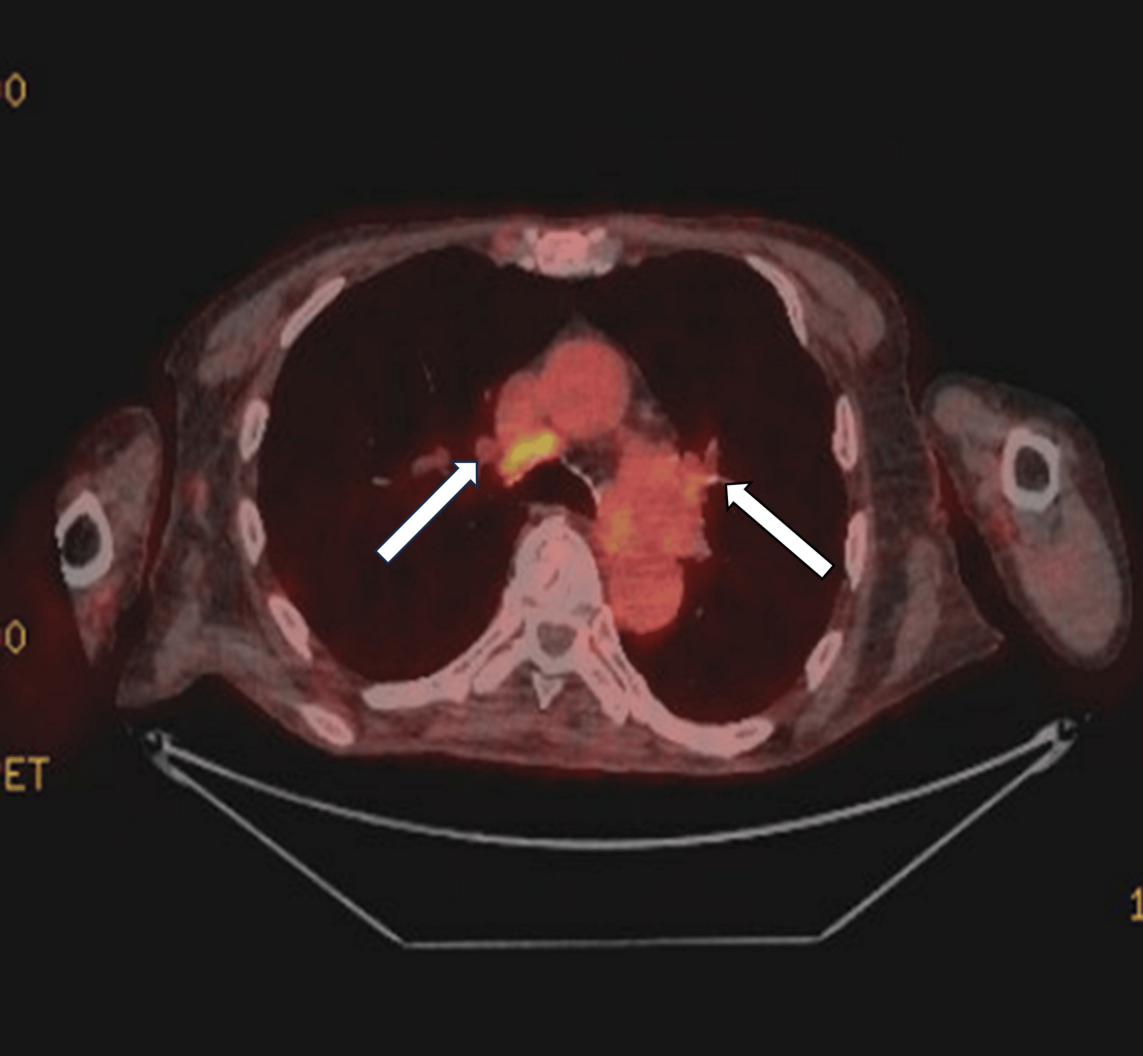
PET CT scan image Mildly fluorodeoxyglucose (FDG) avid bilateral hilar lymphadenopathy was seen. PET: Positron emission tomography.
